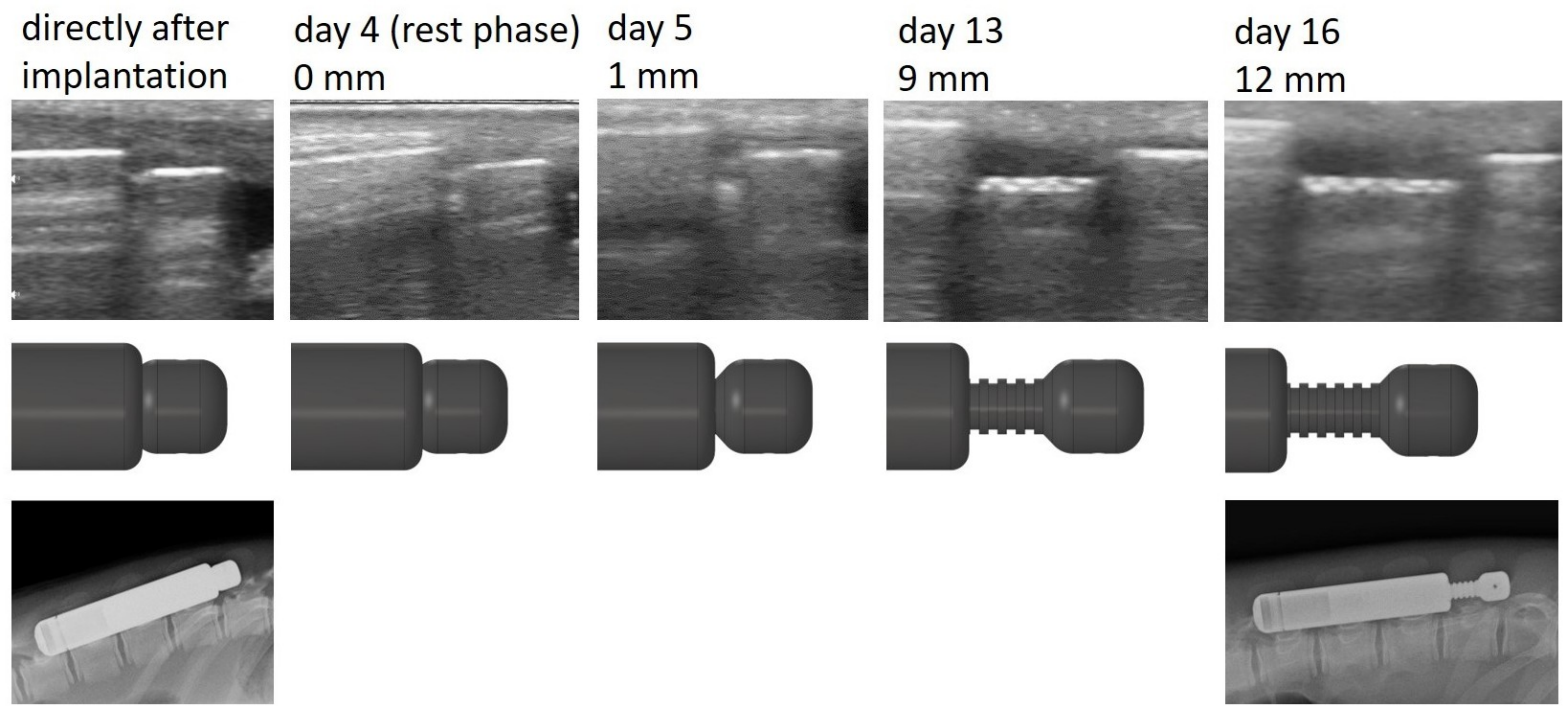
Series of ultrasound scans (upper row) and distraction in scheme (mid row) directly after implantation, on day 4 (last day of rest phase), day 5 (first day of elongation), day 8, day 13 and right before explantation on day 16. Notches with 1 mm width served as a distance indicator in the ultrasound scans. X-ray imaging (bottom row) was performed directly after implantation and before retrieval, both with anesthesia.
